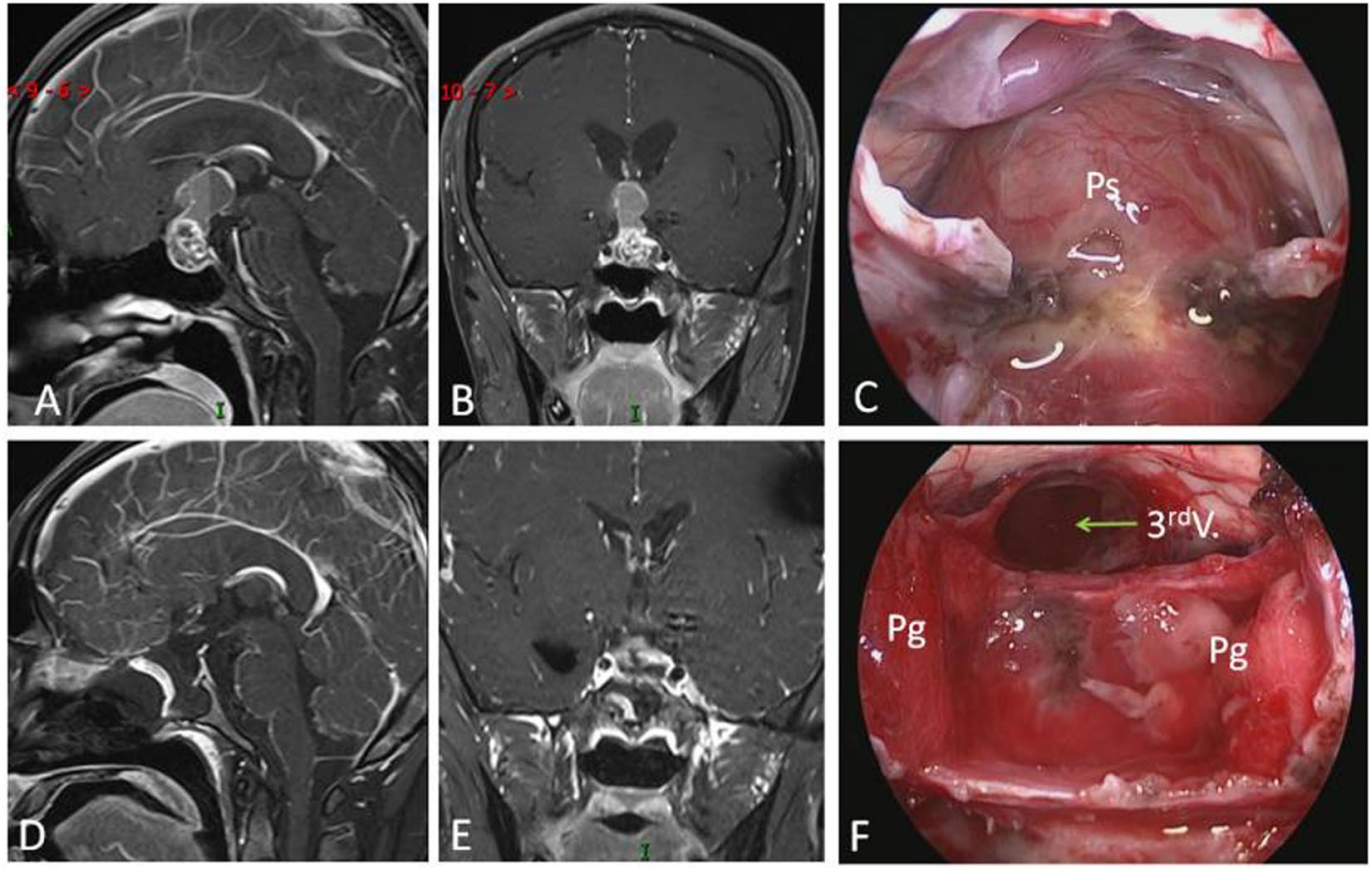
Example of a Central type Cp grows longer in vertical direction. (A and B) Sagittal (A) and Coronal (B) postcontrast T1-weighted MR images showing that tumor grows up to the third ventricle and down to the intrasellar region through pitiutary stalk. (C and F) Intraoperative photographs showing that tumor grew within and along the stalk and down to the intrasellar region (C). The third ventricle floor was destroyed, residual pituitary gland pushed and compressed by the tumor could be seen after tumor removal (F). (D and E) Sagittal (D) and Coronal (E) postcontrast T1-weighted MR images obtained after GTR demonstrating extensive, safe resection of the tumor. 3^rd^ V., the third ventricle; Pg, pituitary gland.
